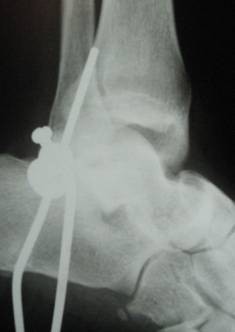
lateral radiograph of the ankle showed total lateral talus dislocation.
